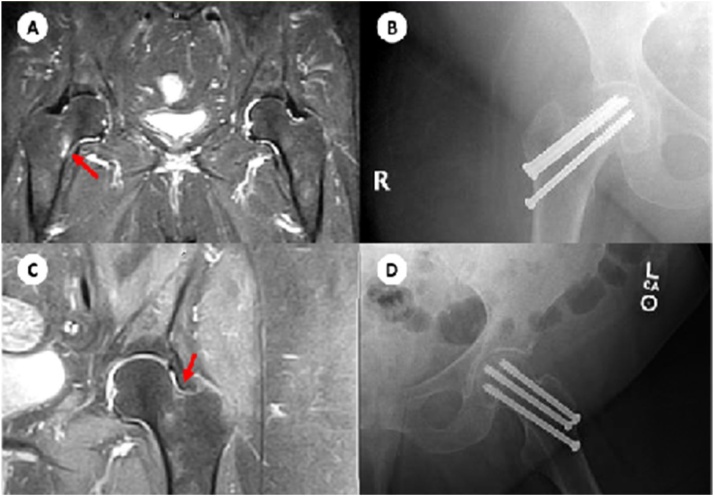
(A) MR study of the pelvis which demonstrates a right femoral neck stress fracture on the compression side type that involves >50% of the cortical bone. (B) Radiograph of the right hip demonstrating postoperative changes status-post closed reduction percutaneous pinning of the femoral neck using three cannulated screws. (C) MR study of the left hip which demonstrates a left femoral neck stress fracture on the tension side that is unicortical. (D) Radiograph of the left hip demonstrating postoperative changes status-post closed reduction percutaneous pinning of the femoral neck using three cannulated screws.
